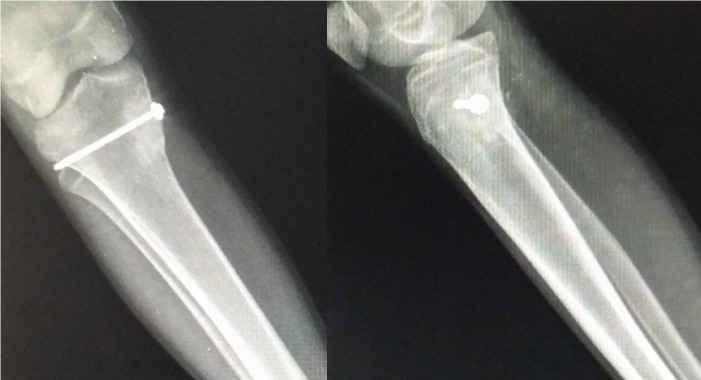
Follow-up radiograph at one year The images show good incorporation of the graft and no signs of recurrence.
